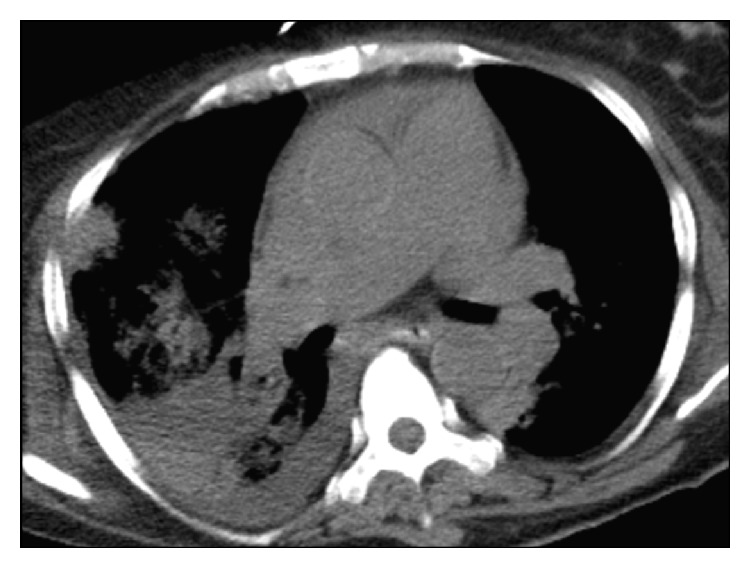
CT scan of the chest, depicting wedge shaped large pulmonary infarct.
